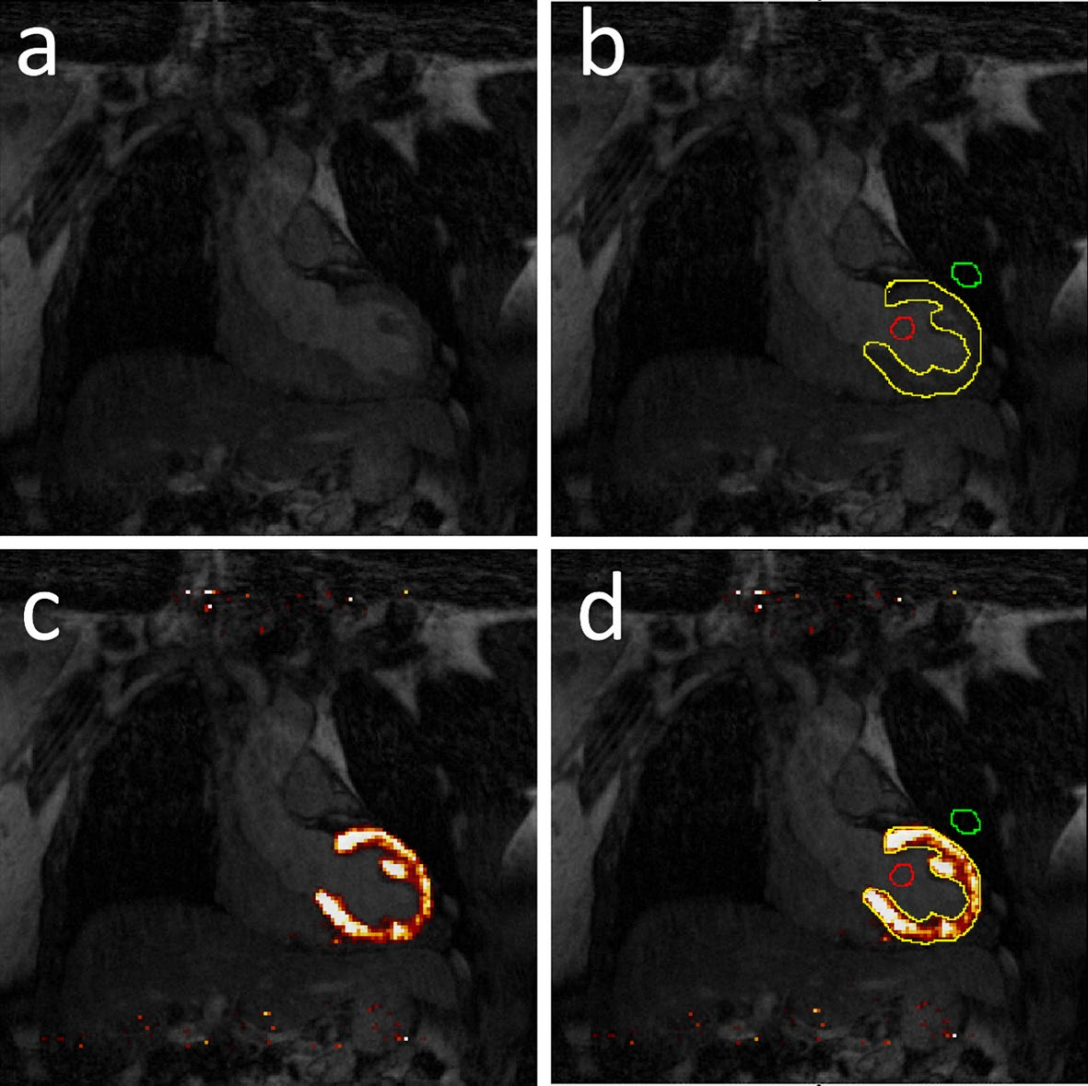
MR and ^18^F-FDG PET/MR images of the myocardium. a CMRA image, b CMRA image with the volumes of Interest (VOIs) that encompasses the left ventricular wall (yellow), a VOI in the middle of the left ventricular cavity (red) and a VOI in the lung close to the myocardium (green) c shows a color overlay of the PET image projected on the CMRA image. d PET/MR image with the three VOIs
